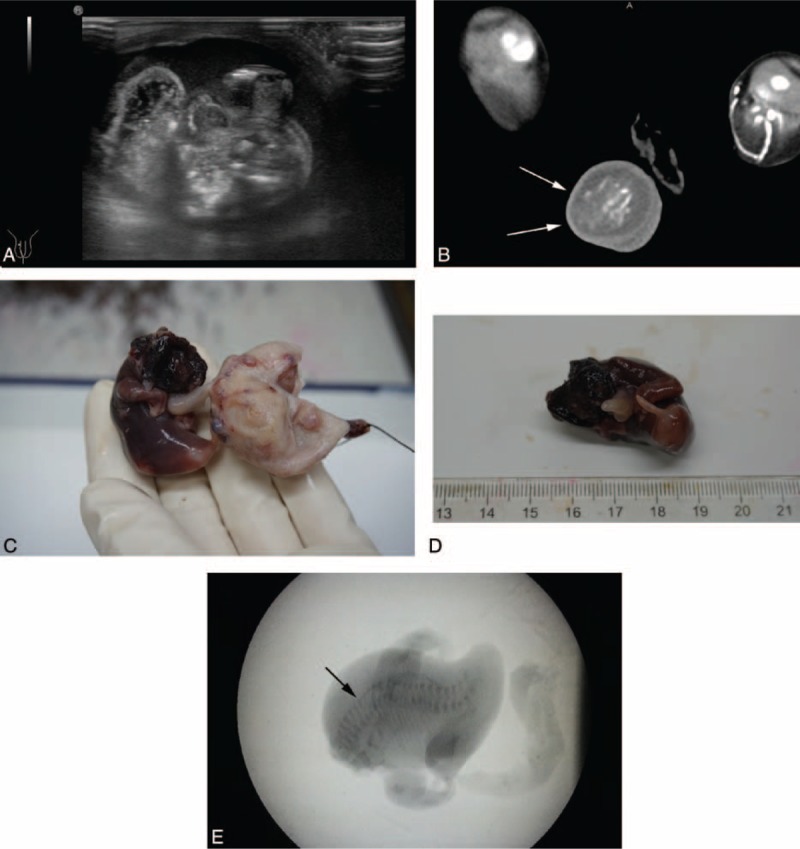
Scrotal FIF in a 20-day-old male neonate. (A) Ultrasound featuring a fetiform mass in the scrotal sac. (B) CT scan demonstrating a well-defined mass, measuring 4.5 × 4.0 cm, surrounded by a fluid-filled sac containing bony elements resembling the vertebral axis and the iliac bones (arrow head). (C and D) Upon incision, the mass exhibits a pinkish fetiform morphology, with tiny male external genitalia at one pole. (E) Plain radiograph of the FIF demonstrating vertebral organization (arrowhead). CT = computed tomography, FIF = fetus in fetu.
